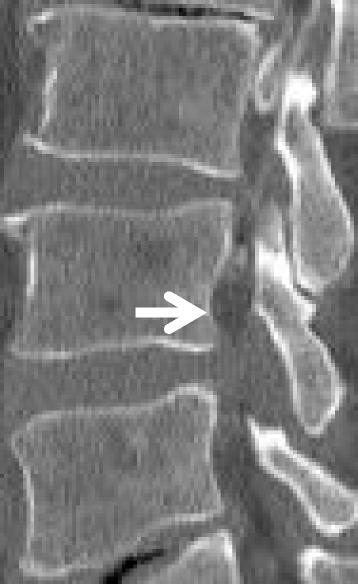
Saucerization of posterior vertebral body on computed tomography (CT). Saucerization of posterior vertebral body is defined findings of compression of more than 2 mm than the usual posterior body of a vertebra in the reconstructed sagittal CT view (arrow)
